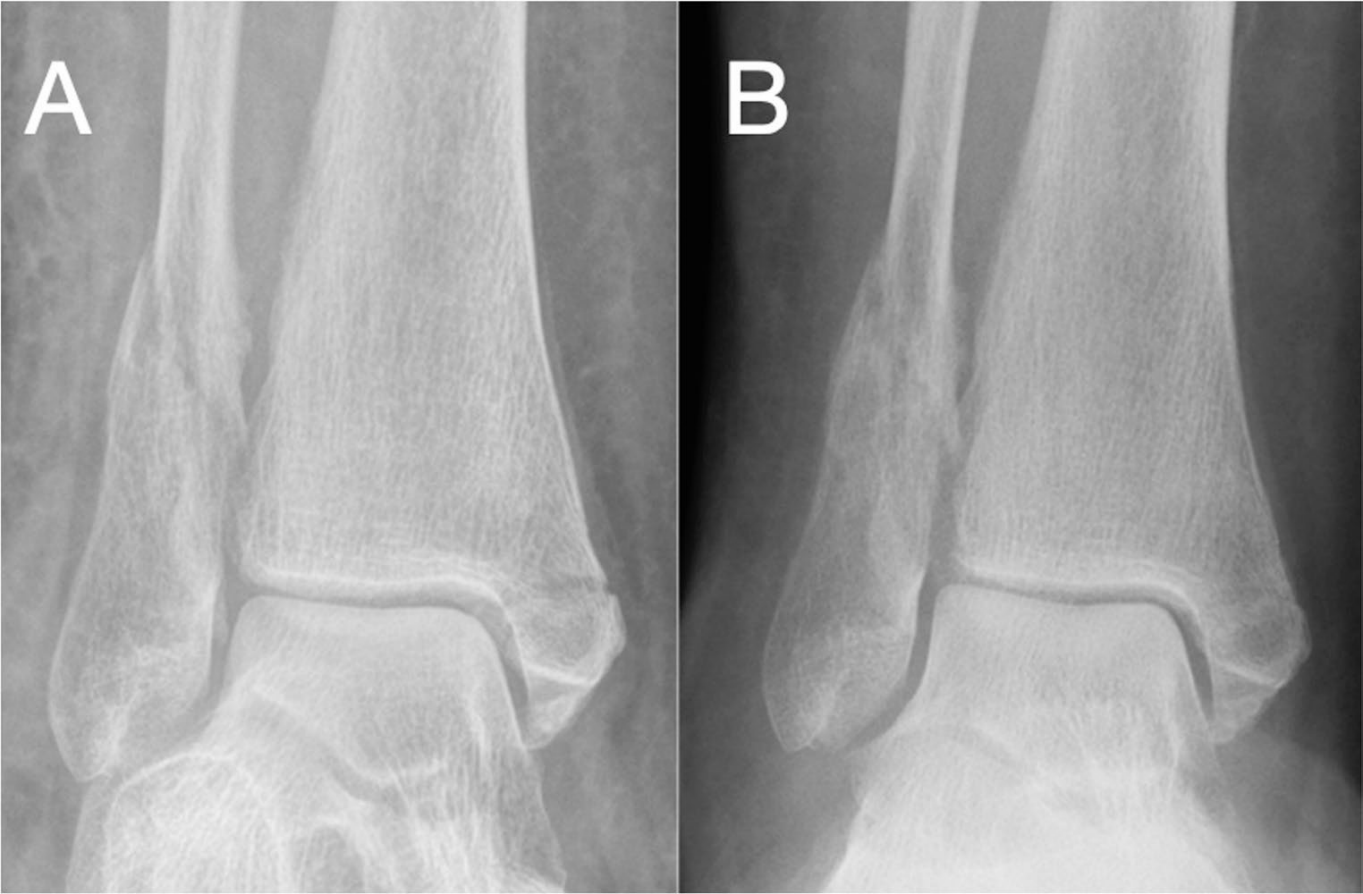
Preoperative (A) and 6 weeks postoperative (B) radiographs of an unstable ankle fracture treated in a cast
